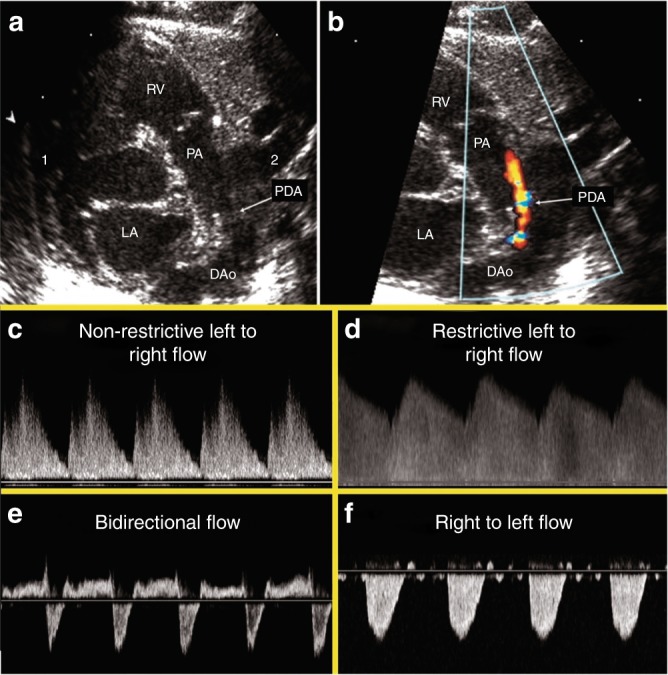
PDA 2D, color Doppler image and Doppler flow patterns. The top panels demonstrate the PDA in 2D (a) and color Doppler (b). c Pulsatile or non-restrictive pattern: characterized by a left to right (LtR) shunt with an arterial waveform and high peak systolic velocity: end-diastolic velocity ratio. d Restrictive pattern: characterized by high systolic and diastolic velocity, and low peak systolic velocity: end-diastolic velocity ratio. e Bidirectional pattern: elevated pulmonary pressures equal to or near systemic. f Right to left (RtL) flow: supra-systemic pulmonary pressures
